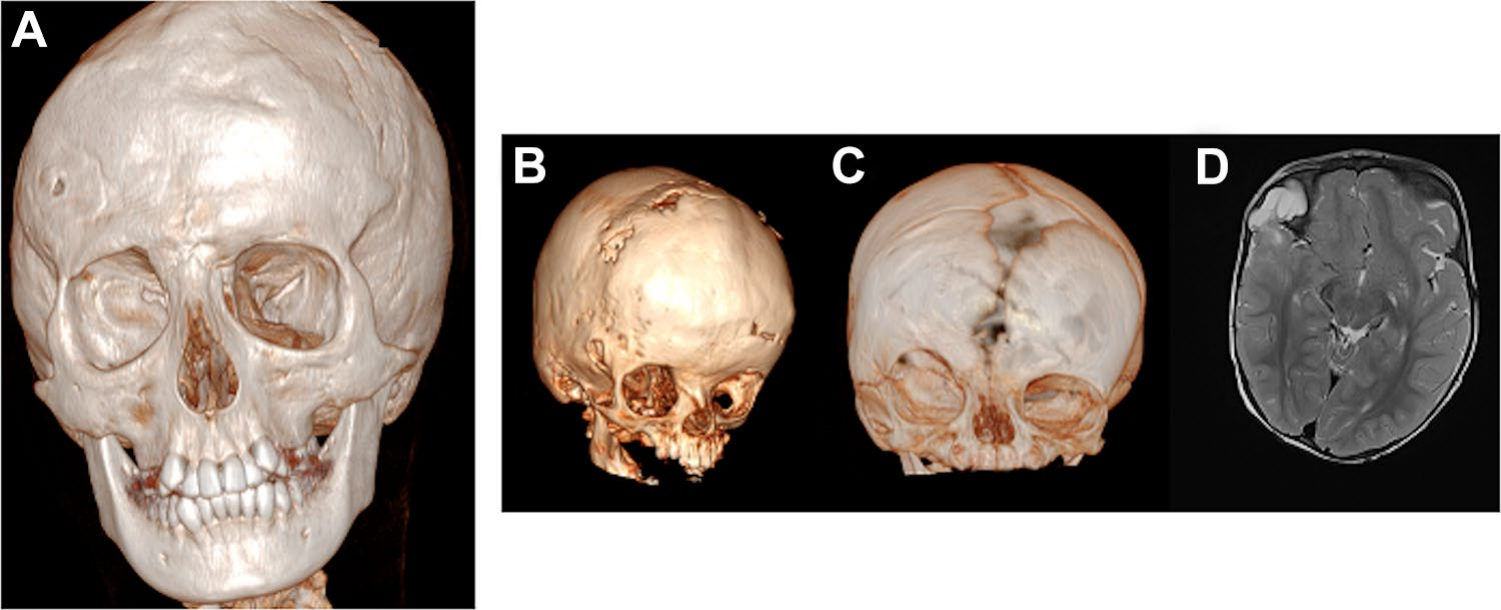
A A 3D reconstruction of a CT scan performed to evaluate the bony asymmetry after a surgical remodelling of an anterior plagiocephaly. A flattening of the right side of the frontal bone was confirmed. B, C 3D reconstructions of the postoperative results after plagiocephaly remodelling confirming a residual asymmetry of the frontal bone. D An axial T2-weighted MRI confirming the presence of an encephalocele, secondary to a dural tear accidentally performed during the first surgery. A second surgery performed 2 years after the first one allowed to address the frontal flattening and the treatment of the encephalocele
